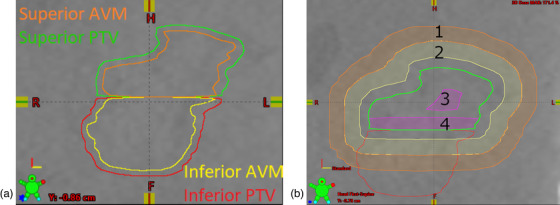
Target and control structures used in arteriovenous malformation (AVM) and PTV volume staging treatment planning in Eclipse. (a) AVM and PTV structures used for patient 8 are shown on a coronal slice of the planning computed tomography (CT) in the specified colors. The AVM volume was divided through the thinner section of the volume to minimize the junction area. (b) Control structures used for the superior stage of patient 8 are shown on the coronal plane of the patient's planning CT. The PTV for the superior stage is shown in green. Structures 1 and 2 are shells created from the PTV at 2 and 5 mm distance from its edge and were used to control the dose falloff from the PTV. Structure 3 was used to keep maximum doses away from the edges of the target, and structure 2 was used to control the dose at the junction of the two stages. Similar structures were also created for the inferior stage.
